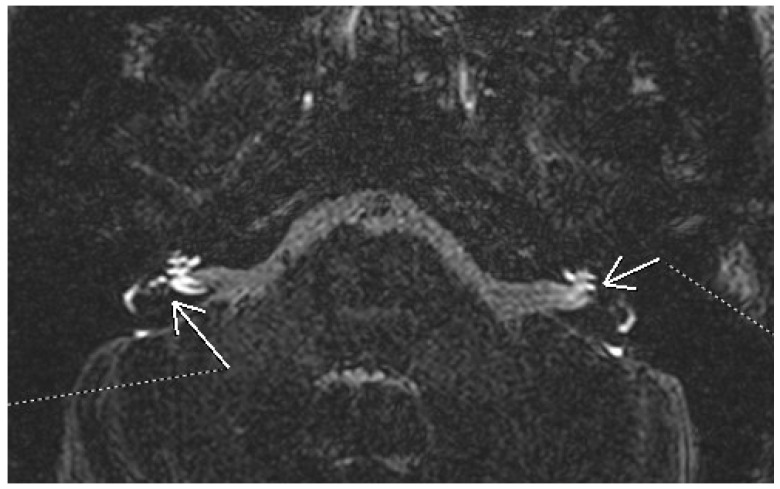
3D FLAIR MRI using a 3T scanner and delayed gadolinium protocol, showing bilateral cochlear and vestibular hydrops.
